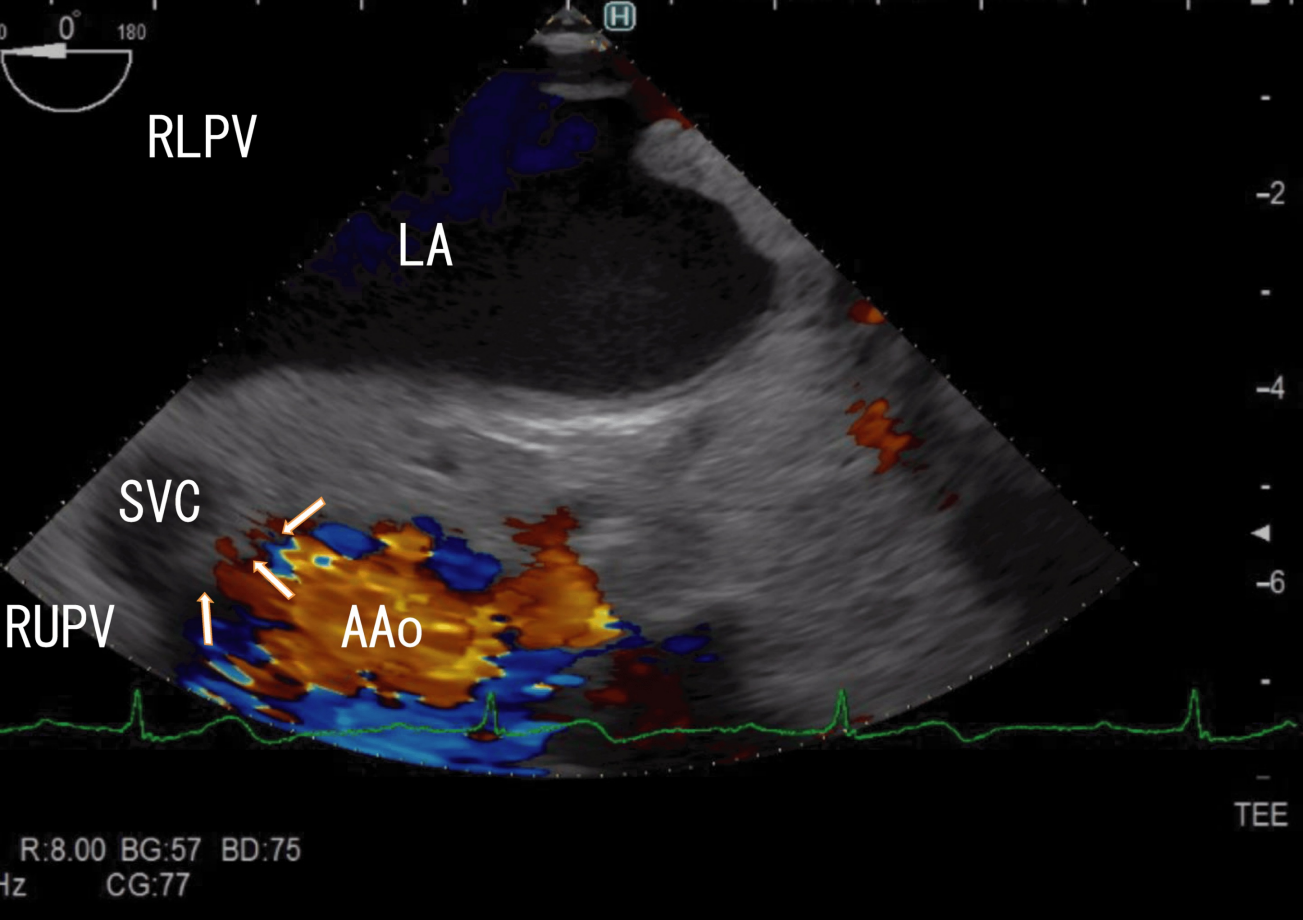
TEE images showing resolving AAo thrombi TEE revealed half of the AAo thrombi in red, blue, and yellow, indicating their resolution. On the upper right side of the AAo, there were a few AAo thrombi (white arrows). TEE: transesophageal echocardiography, AAo: ascending aorta, LA: left atrium, SVC: superior vena cava, RLPV: right lower pulmonary vein, RUPV: right upper pulmonary vein
